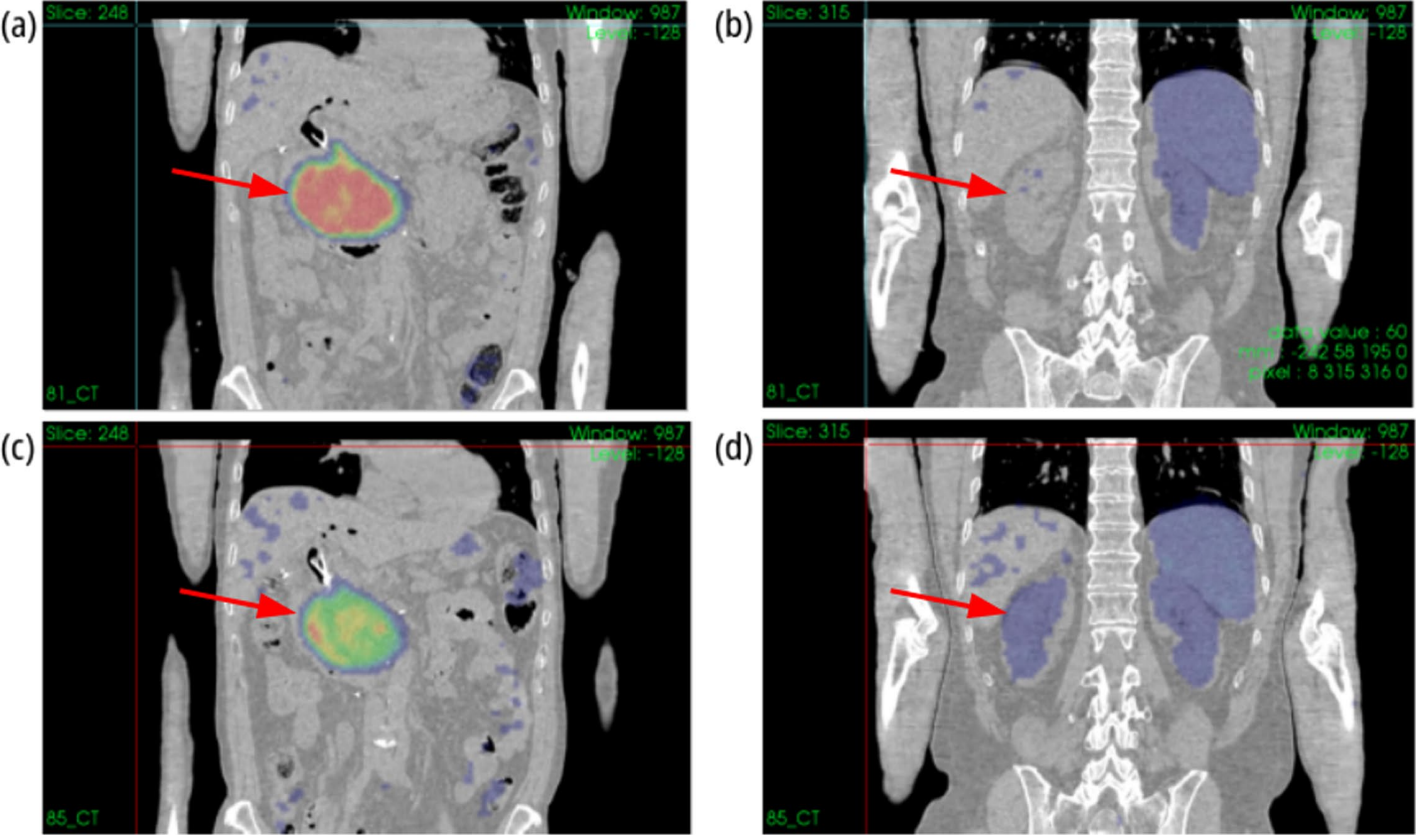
SPECT images superimposed on CT scans for patient 5 of the patient cohort at cycles 1 (a, b) and 4 (c, d) showing the evolution of biodistribution of activity from one cycle to another at the lesion level (a, c) and at the right kidney level (b, d). The same color scale was used for all images
